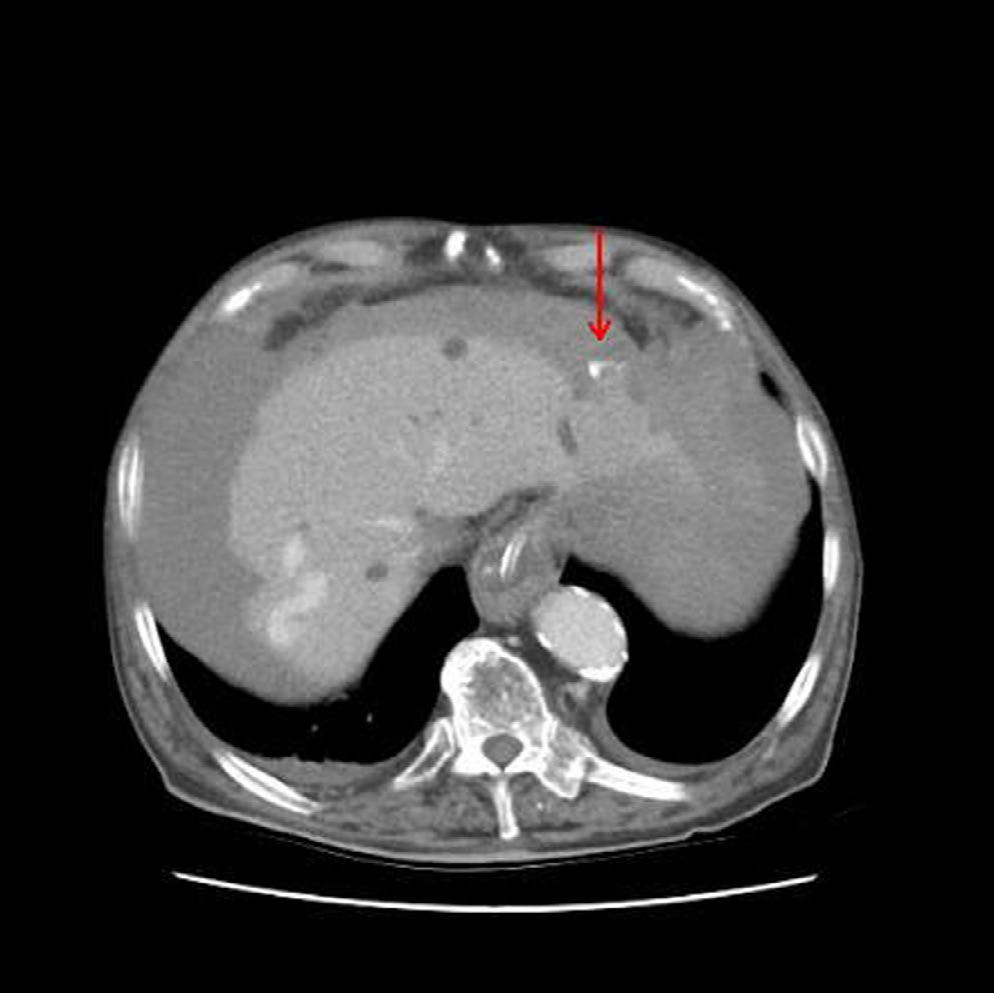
CT revealed no features of pulmonary infection, liver surface nodularity, mild ascites at Morrison and Douglas pouch with 35–45 HU density, a persistent hypoattenuating in all phases peripheral segment III nodule with active intravenous contrast extravasation (red arrow), dilated vena cava, no signs of portal hypertension, no intraperitoneal varices, and no free intraperitoneal air
